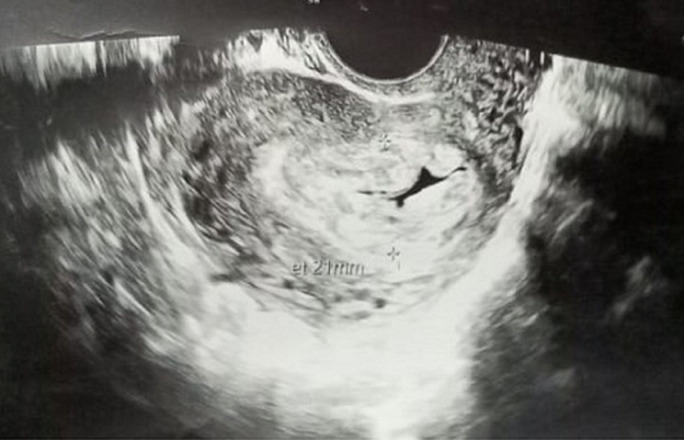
trans abdominal sonography showing endometrial thickness of 21 mm
